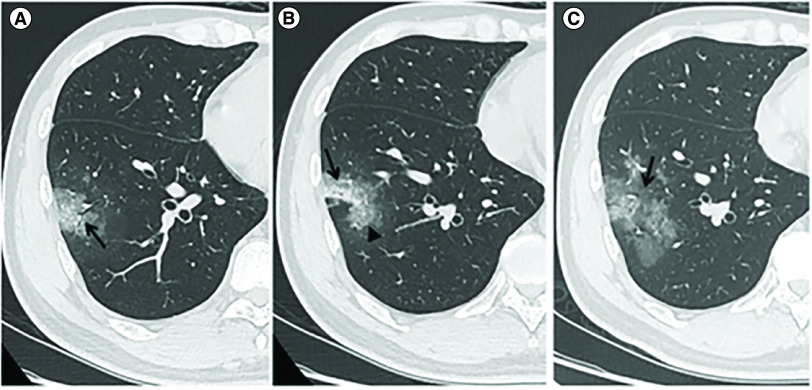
Computed tomography imaging of a case of novel coronavirus pneumonia. (A–C) Chest CT of a 37-year-old male who had a fever for 4 days and showed a common type of novel coronavirus pneumonia. (A & B) Images of the lung window show an irregular shape of GGO combined with a little consolidation opacity located in the lateral basal segment (S9) of the right lower lobe (arrow). Intralobular septal thickening (arrowhead) and air bronchogram sign (arrow) were also present. (C) The follow-up CT image of the lung window 2 days later shows that the density in the middle of the lesion was decreased, but the size of the lesion was increased (arrow). CT: Computed tomography; GGO: Ground-glass opacity.
